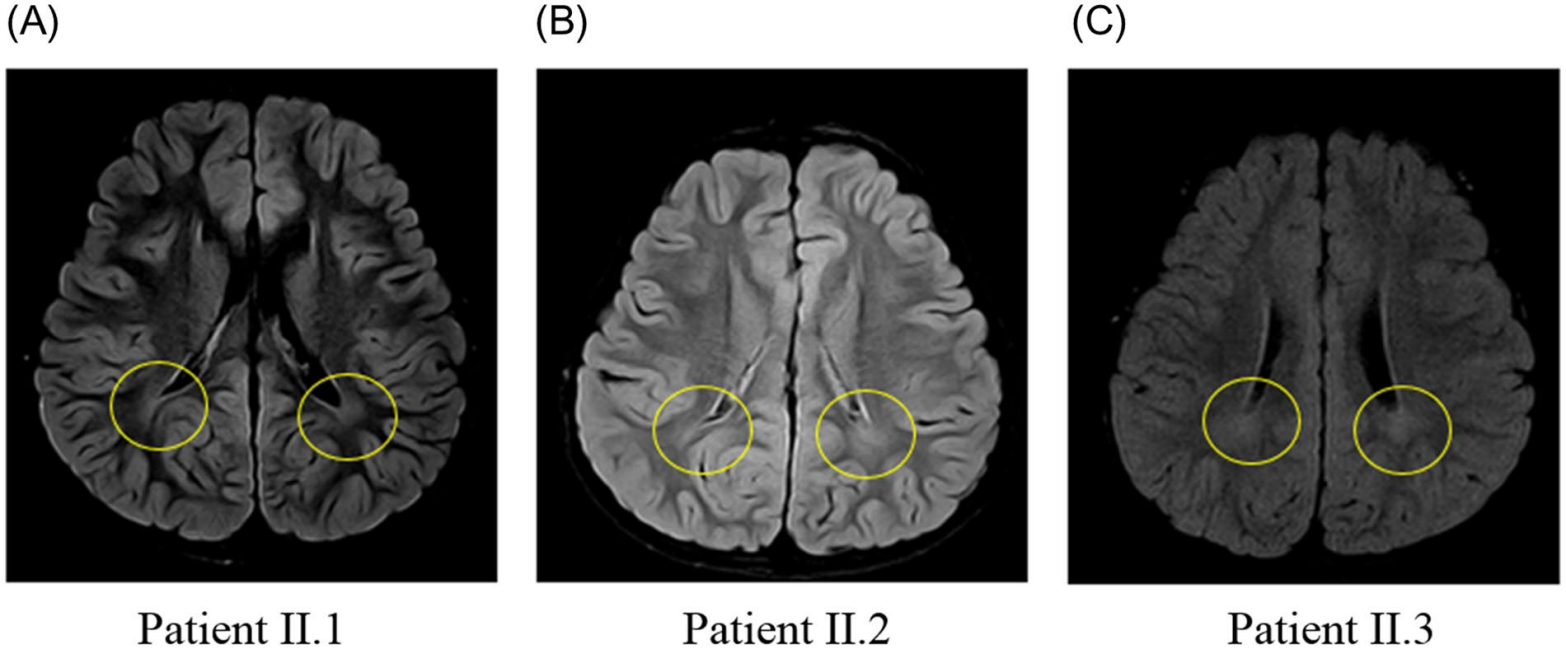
Fluid-attenuated inversion recovery (FLAIR) magnetic resonance image of patients. (A, Patient II.1; B, Patient II.2; C, Patient II.3) FLAIR imaging shows ill-defined lesion of periventricular white matter which is shown in yellow circle.
